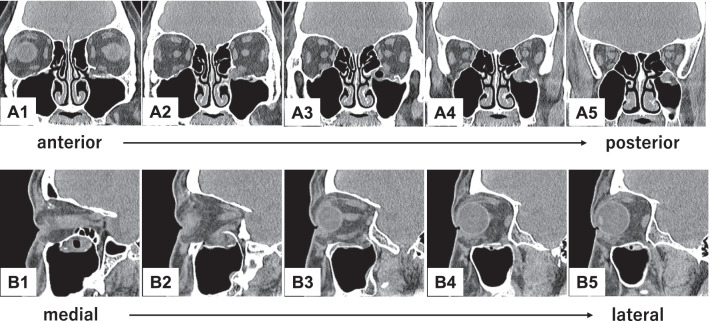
Preoperative computed tomography (CT) images. A1–5 are reformatted coronal CT images in anterior to posterior order. B1–5 are reformatted sagittal CT images in medial to lateral order. Images show an orbital floor fracture that extends to the slope of the posterior end of the orbital floor
